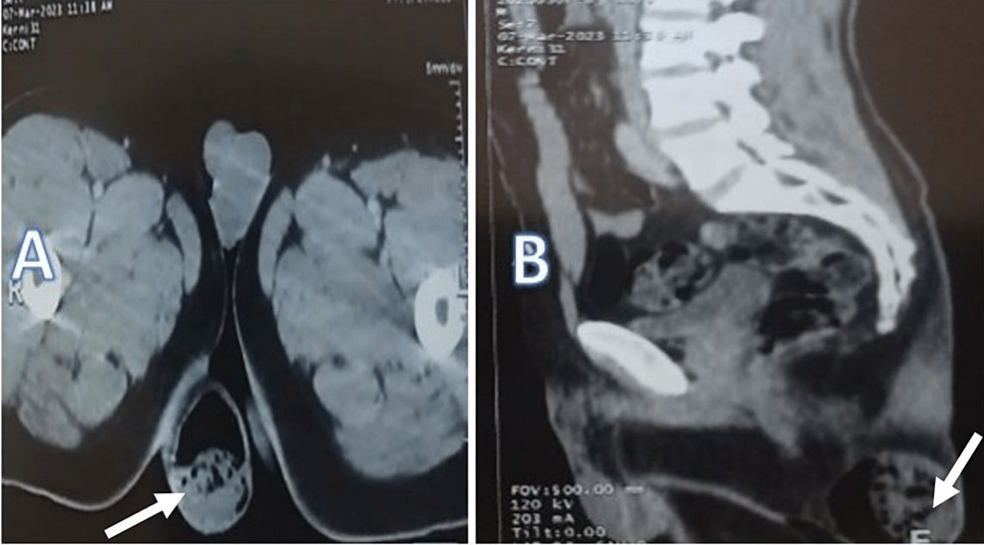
Computed tomography scan showing buttock mass containing air bubbles (white arrow) (A) Sectional axial image; (B) sagittal section
